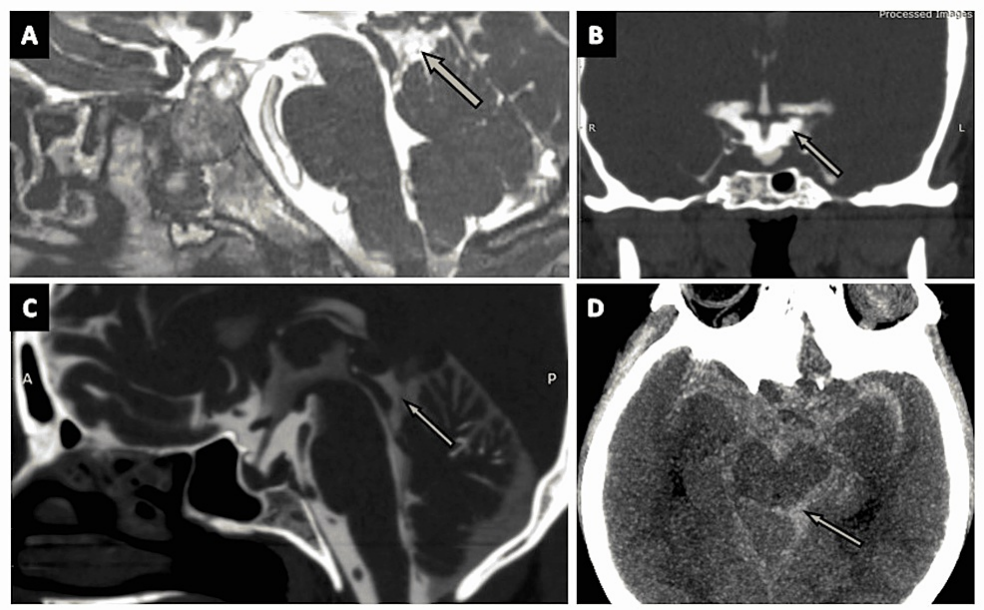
The cisterna ambiens (ambient cistern). (A) The ambient cistern (arrow) as seen on the sagittal section of the routine three-dimensional (3D) constructive interference in steady state (CISS) MRI sequence. (B) and (C) show the ambient cistern (arrows) in the coronal and sagittal planes of CT cisternography, respectively, in a case CSF rhinorrhoea. (D) The ambient cistern (arrow) as seen on the axial section of CT brain (positive contrast) in a case of subarachnoid hemorrhage (SAH).
